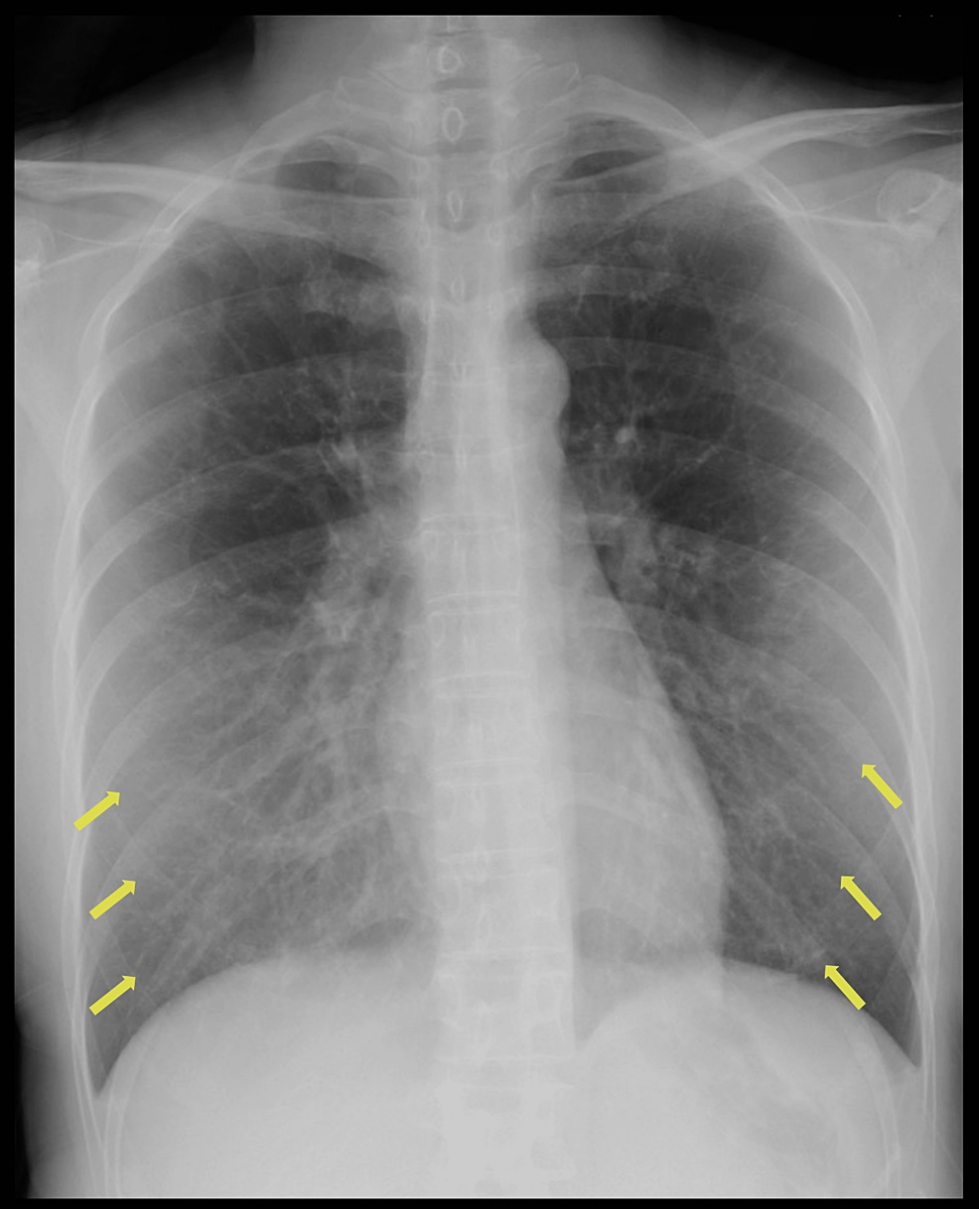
Chest radiographs showing frosted and granular shadows predominantly in the bilateral lower lung fields.
